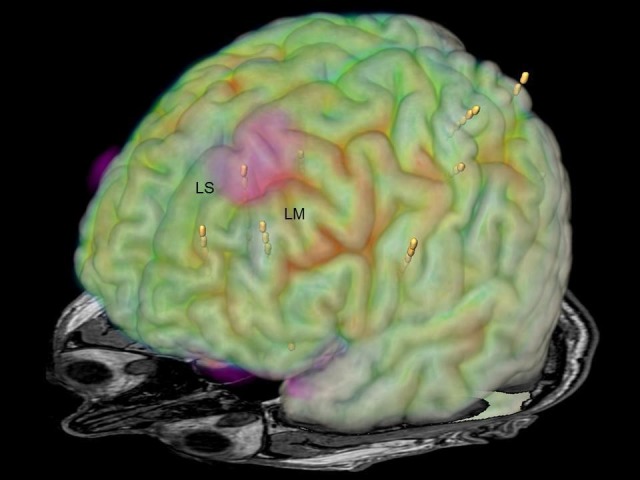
Image fusion of MRI, FDG-PET, DTI-MRI, and depth electrodes Image fusion of MRI, FDG-PET, reduced U-fiber density in DTI-MRI (marked red), and localization of stereotactically implanted depth electrodes (yellow dots). LS: left superior frontal gyrus; LM: left middle frontal gyrus; MRI: magnetic resonance imaging; FDG-PET: fluorodeoxyglucose positron emission tomography; DTI: diffusion tensor imaging
